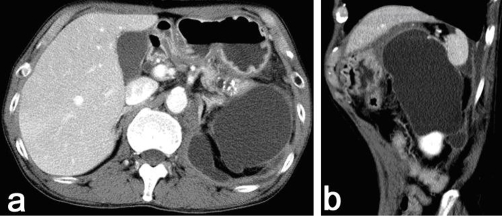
After 4 months conservative treatment CT scan demonstrated the cyst increased in size to 7 cm diameter on horizontal section (a) and 11 cm in the cranio-caudal direction (b).
